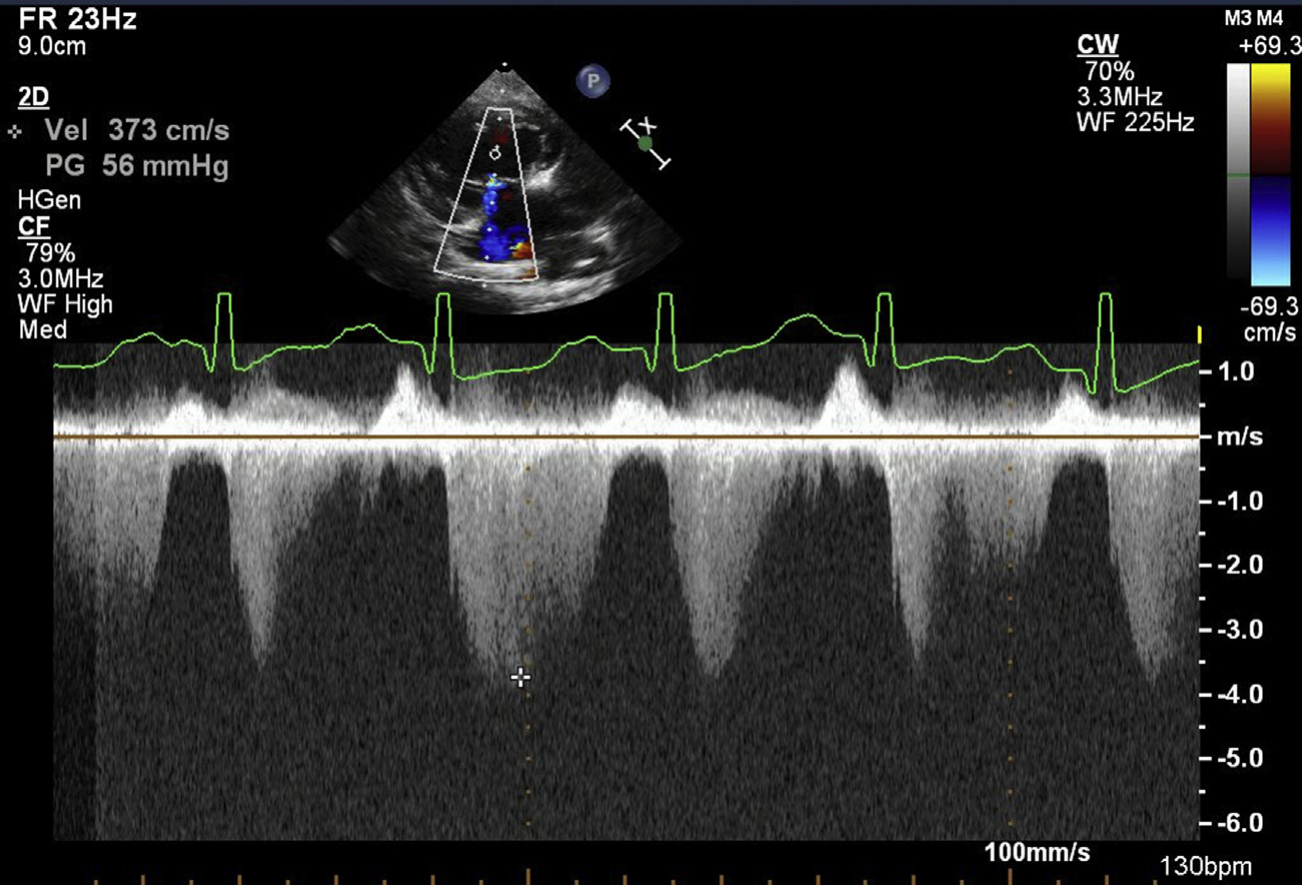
Continuous-wave Doppler assessment of tricuspid valve regurgitation jet from the RV long-axis view of the left parasternal window with RV systolic pressure estimation. The peak velocity was 3.7 m/sec with a calculated peak gradient of 56 mm Hg.
